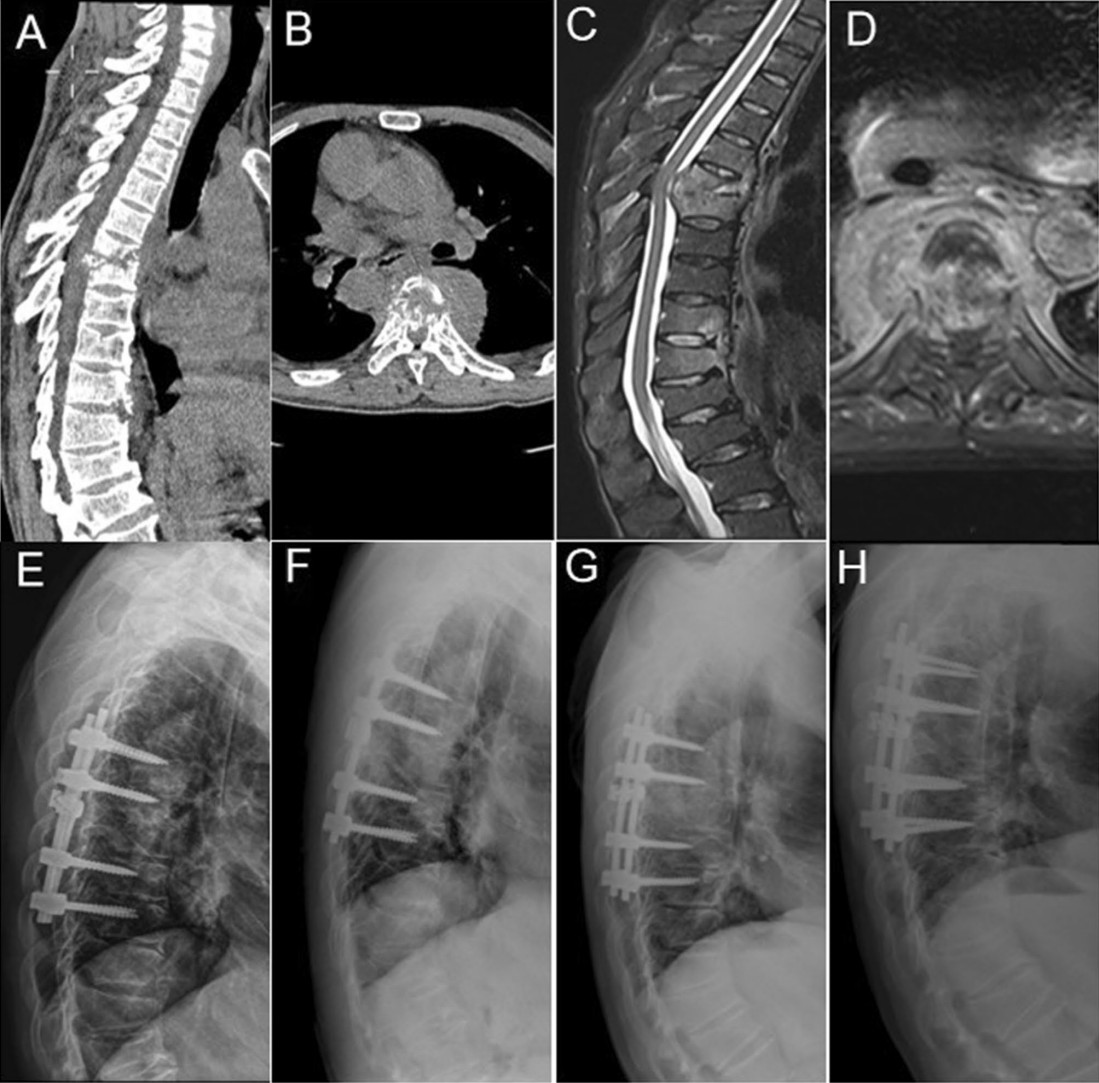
Computed tomography and magnetic resonance imaging (a–d) show T6–T7 vertebral bone destruction, paravertebral abscess formation and spinal cord compression. X-rays taken 3 days post-operatively (e); 6 months post-operatively (f); 12 months post-operatively (g), and; 24 months post-operatively (h).
